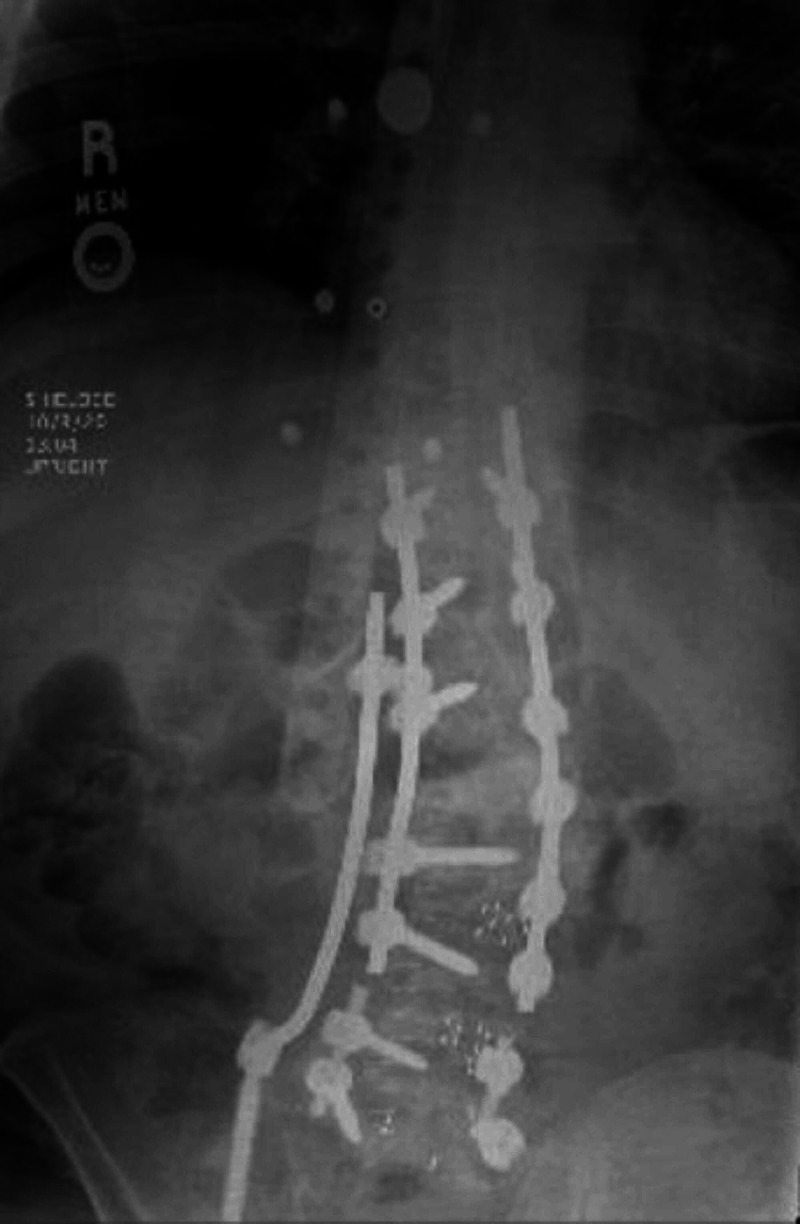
Postoperative standing upright lumbar spine x-rays defining the kickstand rod placement along the patient’s lumbar-iliac junction.
